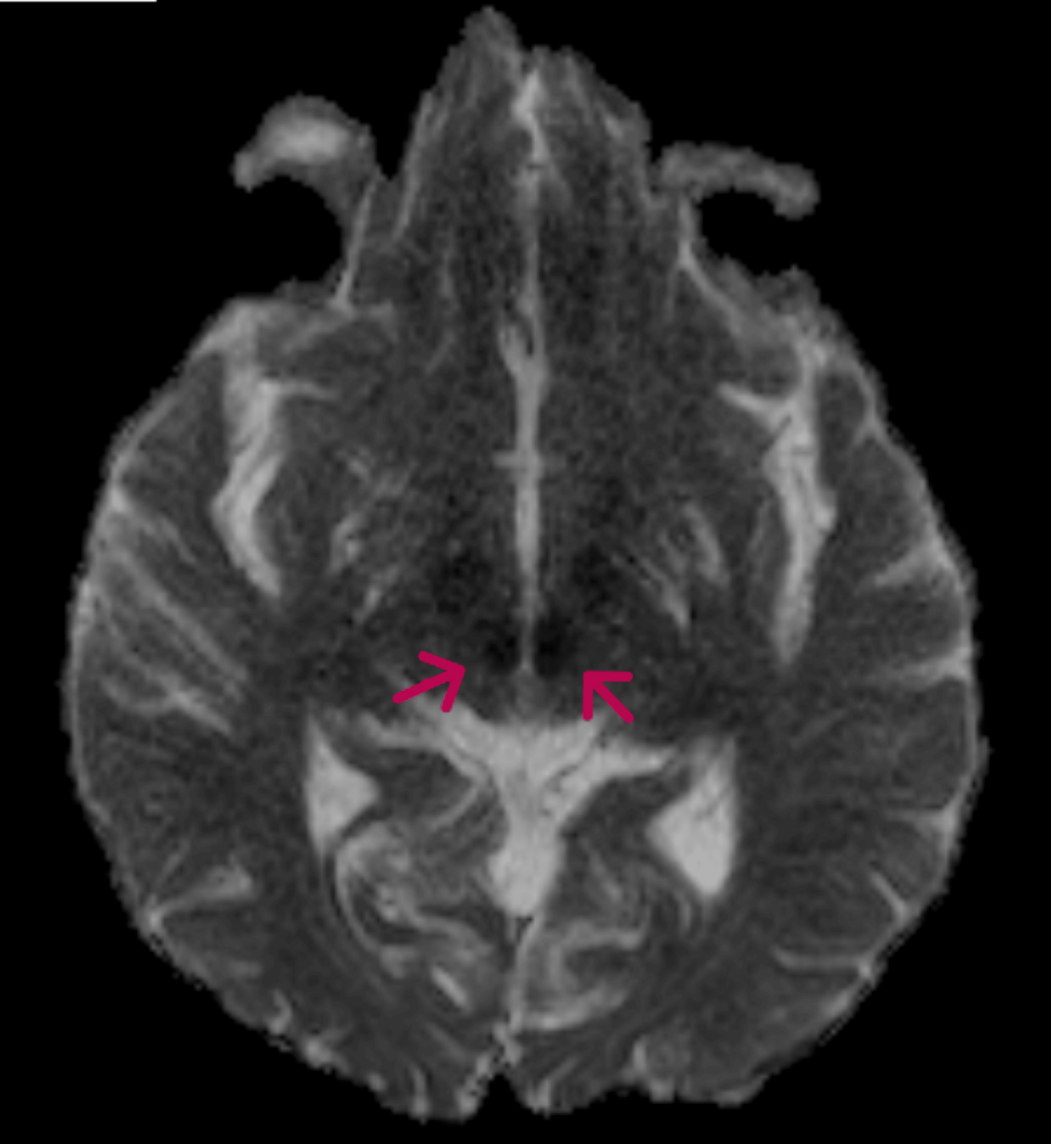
MRI brain (ADC sequence) showing fall in ADC mapping in the bilateral thalami (arrows). ADC: Apparent diffusion coefficient
